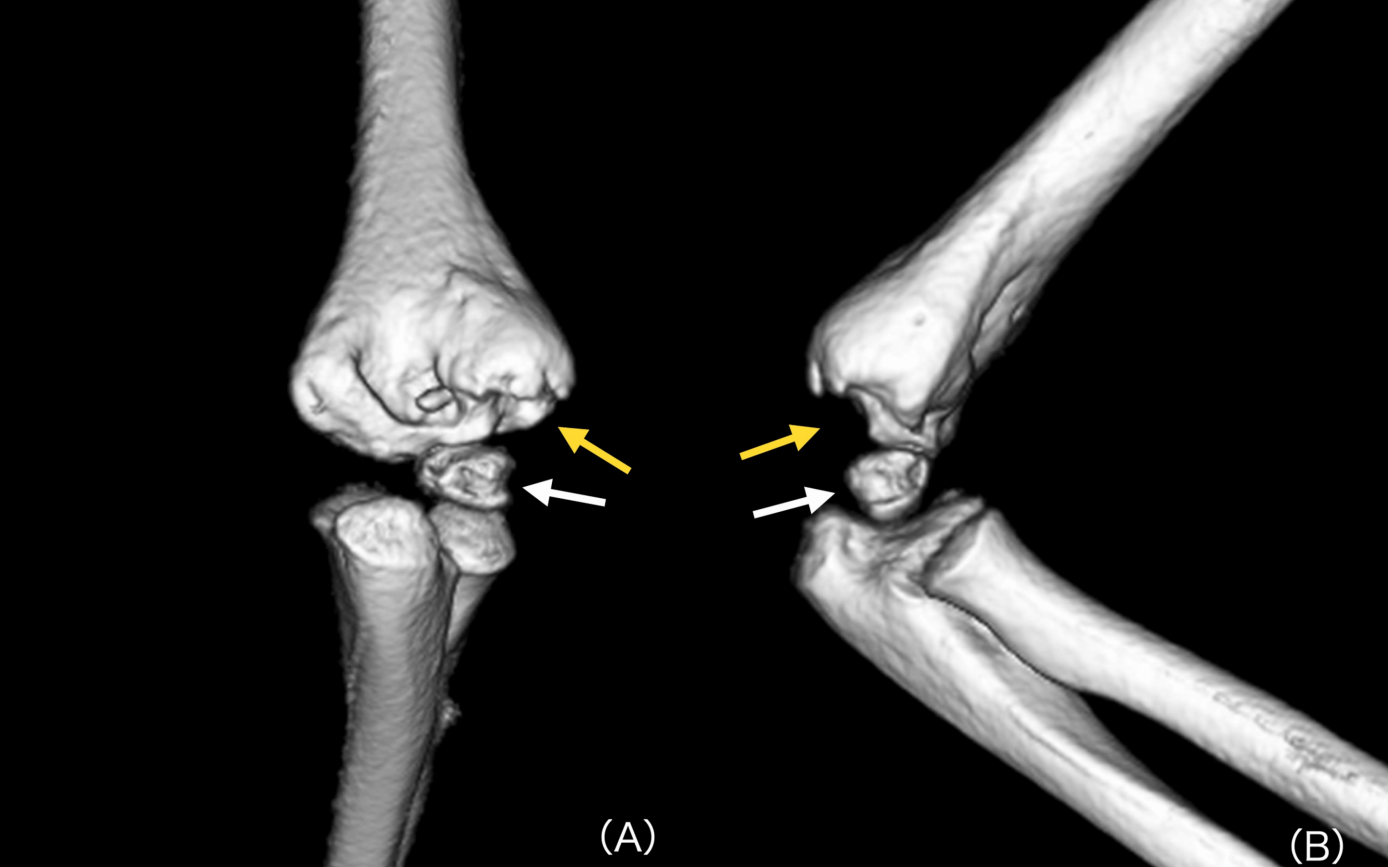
Three-dimensional CT highlighting cortical bone defects Three-dimensional CT reconstruction of the right elbow demonstrating cortical bone defects in the posterolateral distal humerus and capitellum: anteroposterior view (A) and lateral view (B). Yellow arrows indicate the defect in the distal humerus, and white arrows indicate the defect in the capitellum.
